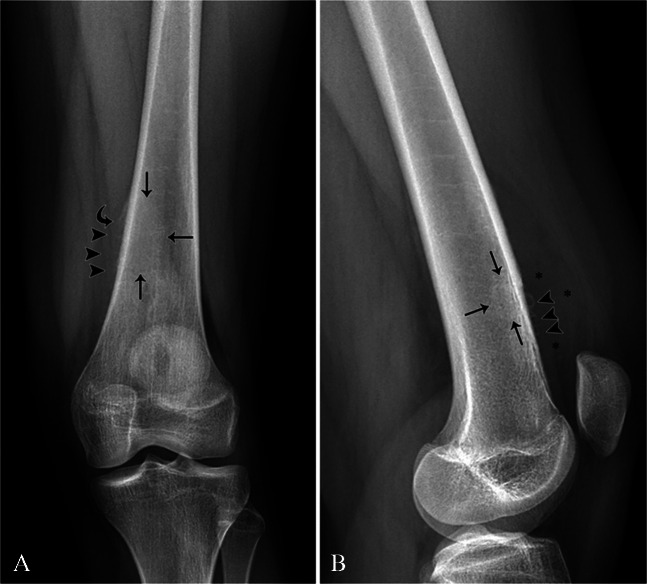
15-year-old female with Ewing sarcoma of the left femur. AP (A) and lateral (B) radiographs demonstrate a subtle mixed sclerotic and lucent lesion (arrows) with a medial Codman's triangle (curved arrow) and anterior "sunburst" periosteal reaction (arrowheads). Extra-osseous extension into the prefemoral space can be seen (*)
